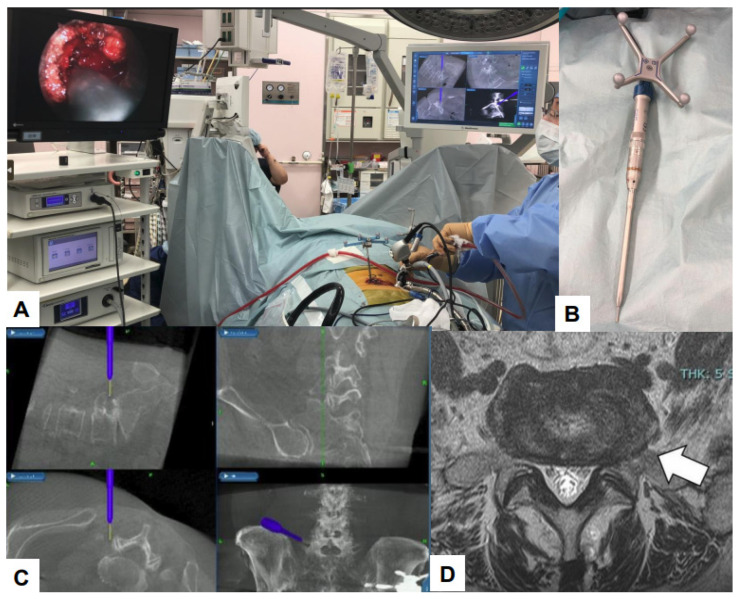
C arm free MED posterolateral approach. (A) Intra-operative image, (B) Navigated high-speed burr, (C) Navigation monitor, (D) Preoperative MRI, A white arrow shows left L5/S1 lateral lumbar disc herniation.
